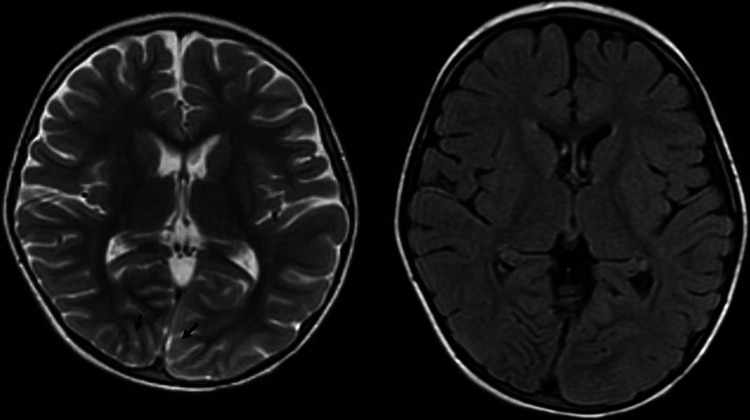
MRI imaging of the brain at the level of the cuneus gyrus demonstrating cortical enhancement of the lingual and cuneus gyri bilaterally with T2-weighted imaging (left). There was no associated restricted diffusion or FLAIR signal abnormality in this region (right).
